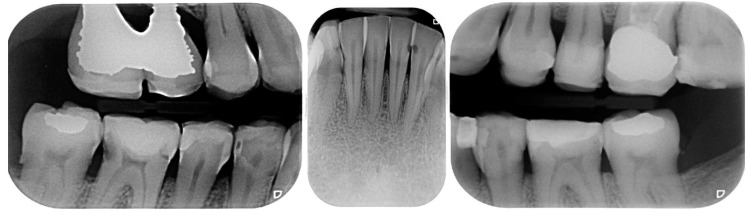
Pre-treatment evaluations by endoral radiographs.
